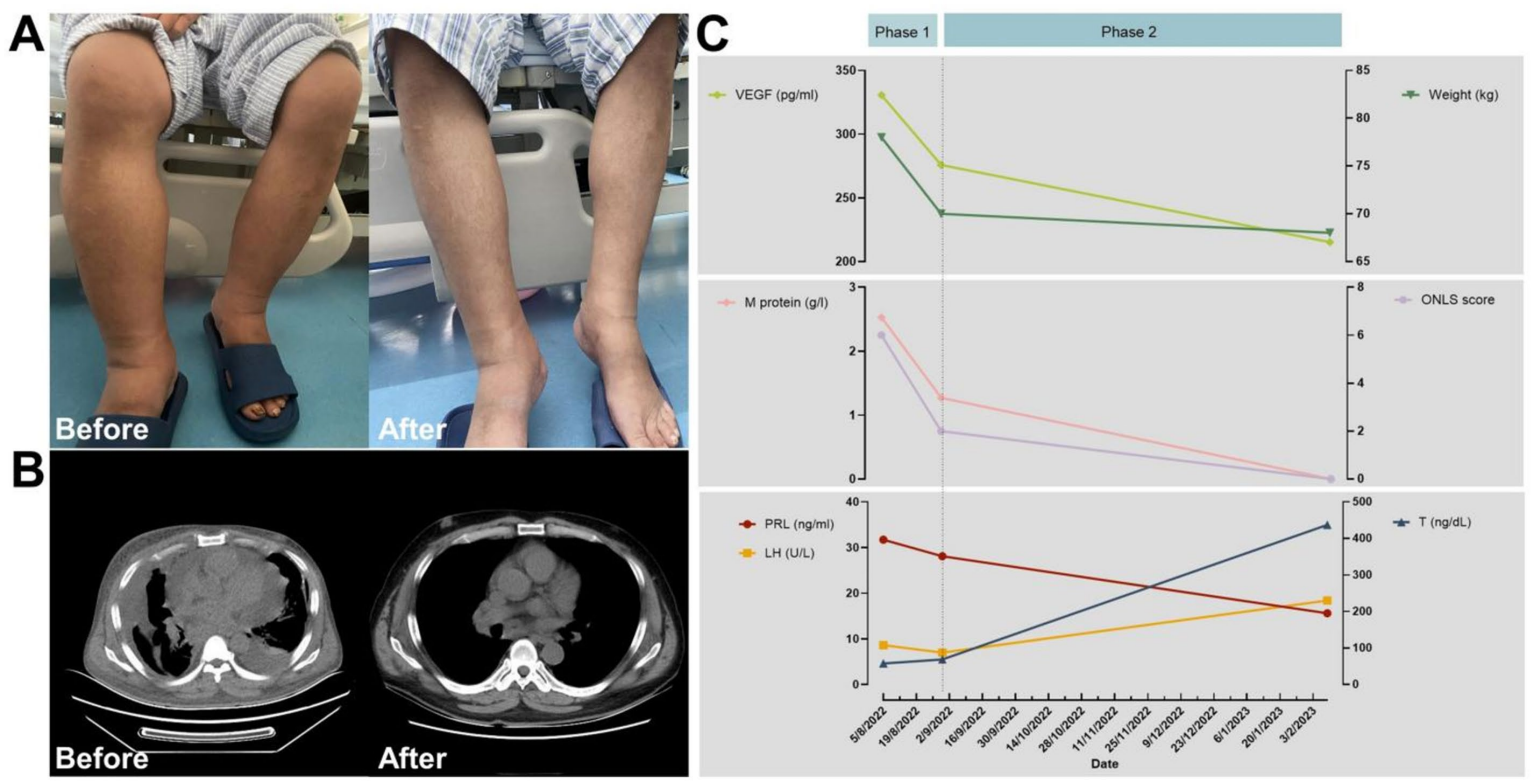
Comparison of patient’s clinical characteristics before and after treatment. (A) Effectiveness of treatment for lower limb edema. (B) Computed tomography showing fluid accumulation in the serous cavities. (C) Treatment course and efficacy; Phase 1: dexamethasone treatment (during the process of diagnosis); Phase 2: three chemotherapy cycles using the Rd. regimen.
